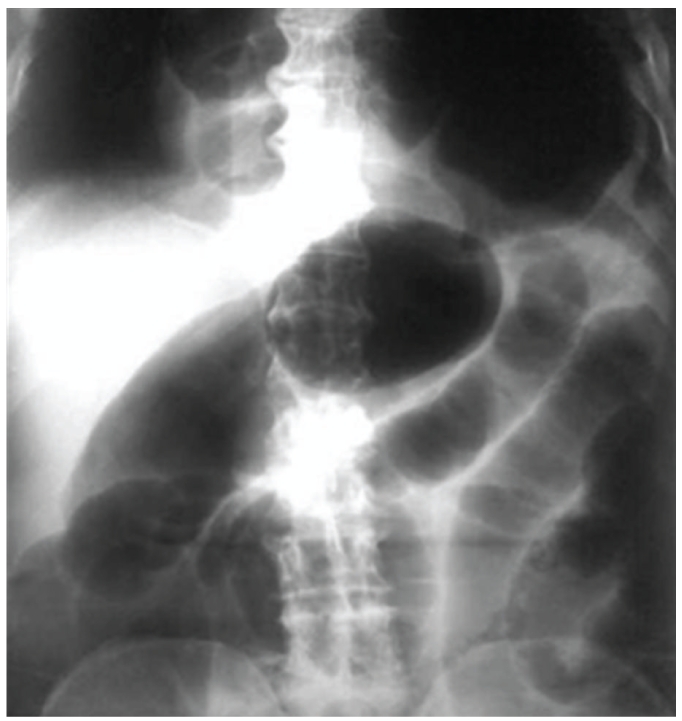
Erect abdominal X-ray reveals a large hiatal hernia with a greatly distended gastric bubble and distended bowel loops.
